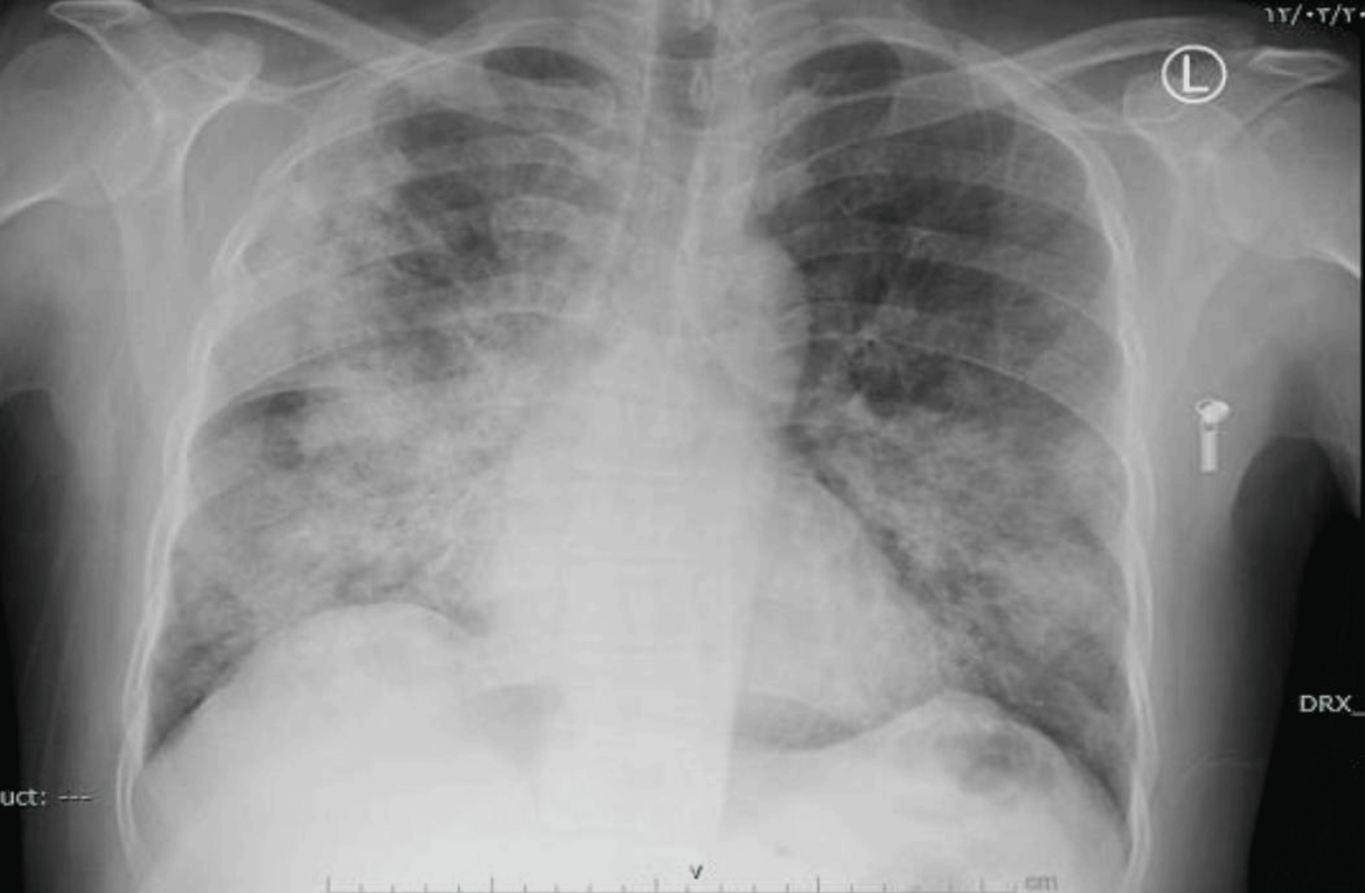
Chest X-ray on admission (Case 1)
